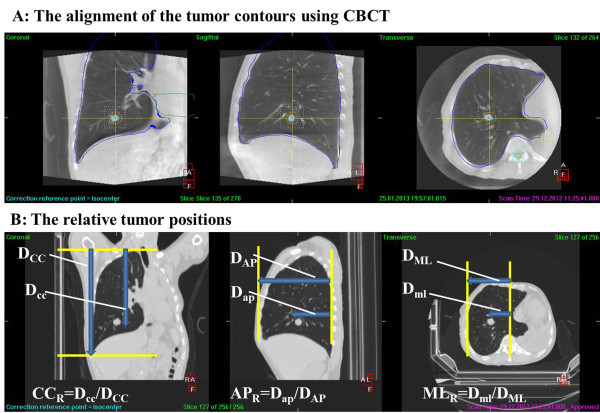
The alignment of the tumor contours using CBCT and the relative tumor position. A: The alignment of the tumor contours using CBCT. The tumor contours in the reference CT image were aligned with those of the CBCT image because the tumor was highly visible in the CBCT image. B: The relative tumor positions defined in the coronal, sagittal and transverse axes.
